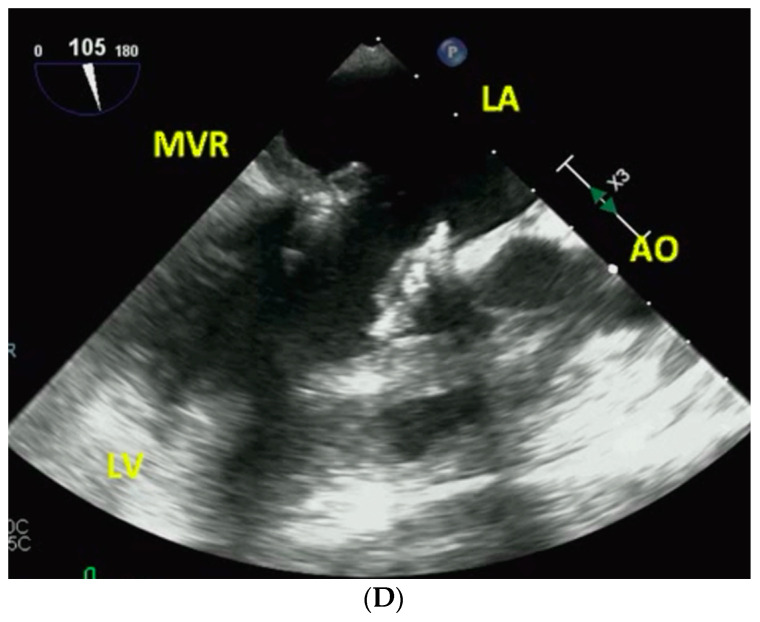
(A–D), Video S2. 58-year-old female with severe mitral valve stenosis following mitral annuloplasty ring implantation 4 years previously (Case 8). Pre-procedure. Two-dimensional transesophageal echocardiography (A). Shows a large TH (arrow) attached to the LA body wall adjacent to AO in A. Spontaneous contrast echoes suggestive of a low flow state are also noted adjacent to the TH. Live/real-time three-dimensional transesophageal echocardiography (B). The large TH attached to the LA body viewed in different cutting planes is demonstrated in B together with spontaneous contrast echoes. Areas of echolucencies noted within the thrombus are consistent with thrombus lysis. During Procedure. Live/real-time three-dimensional transesophageal echocardiography (C, Video S2). The arrow in C points to the catheter deployment device passing through the ring (R) and avoiding the thrombus in full volume mode (Video S2). Post-procedure. Two-dimensional transesophageal echocardiography (D). Demonstrates the new prosthesis in the fully open position in D. Abbreviations as in previous Figures.
